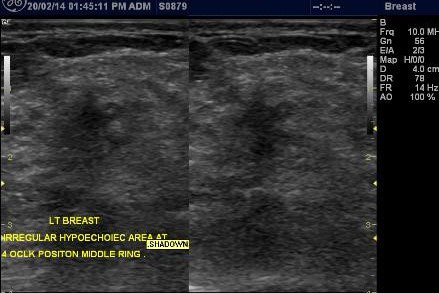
Ultrasound of the breast in orthogonal views showing a spiculated, poorly circumscribed, hypoechoic mass with posterior acoustic shadow in the left breast.
